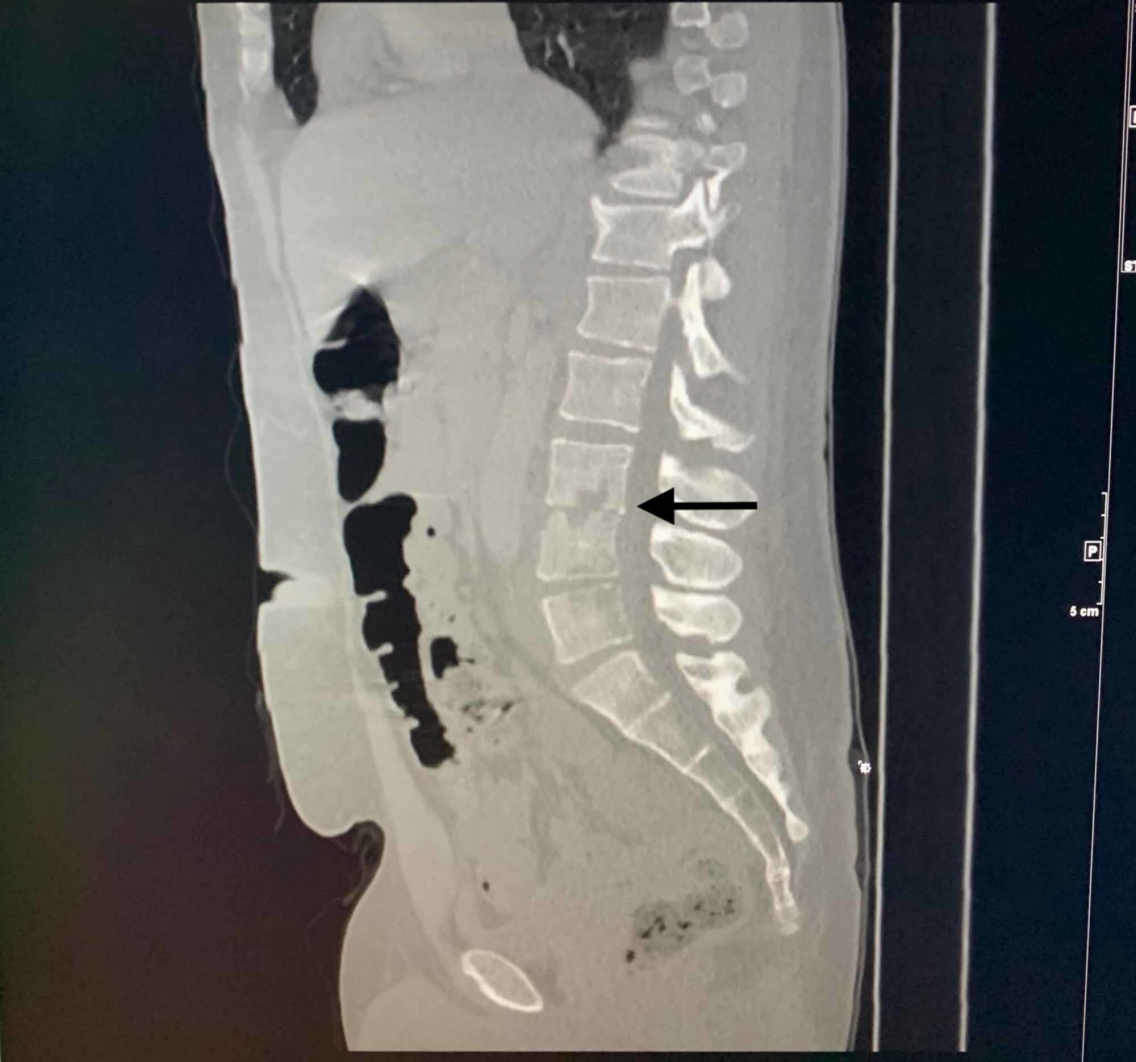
CT of the abdomen and pelvis with contrast showing marked irregularity of the endplates at the L3-L4 level and mild irregularity of the endplates at the L4-L5 level suggestive of osteomyelitis/discitis.
